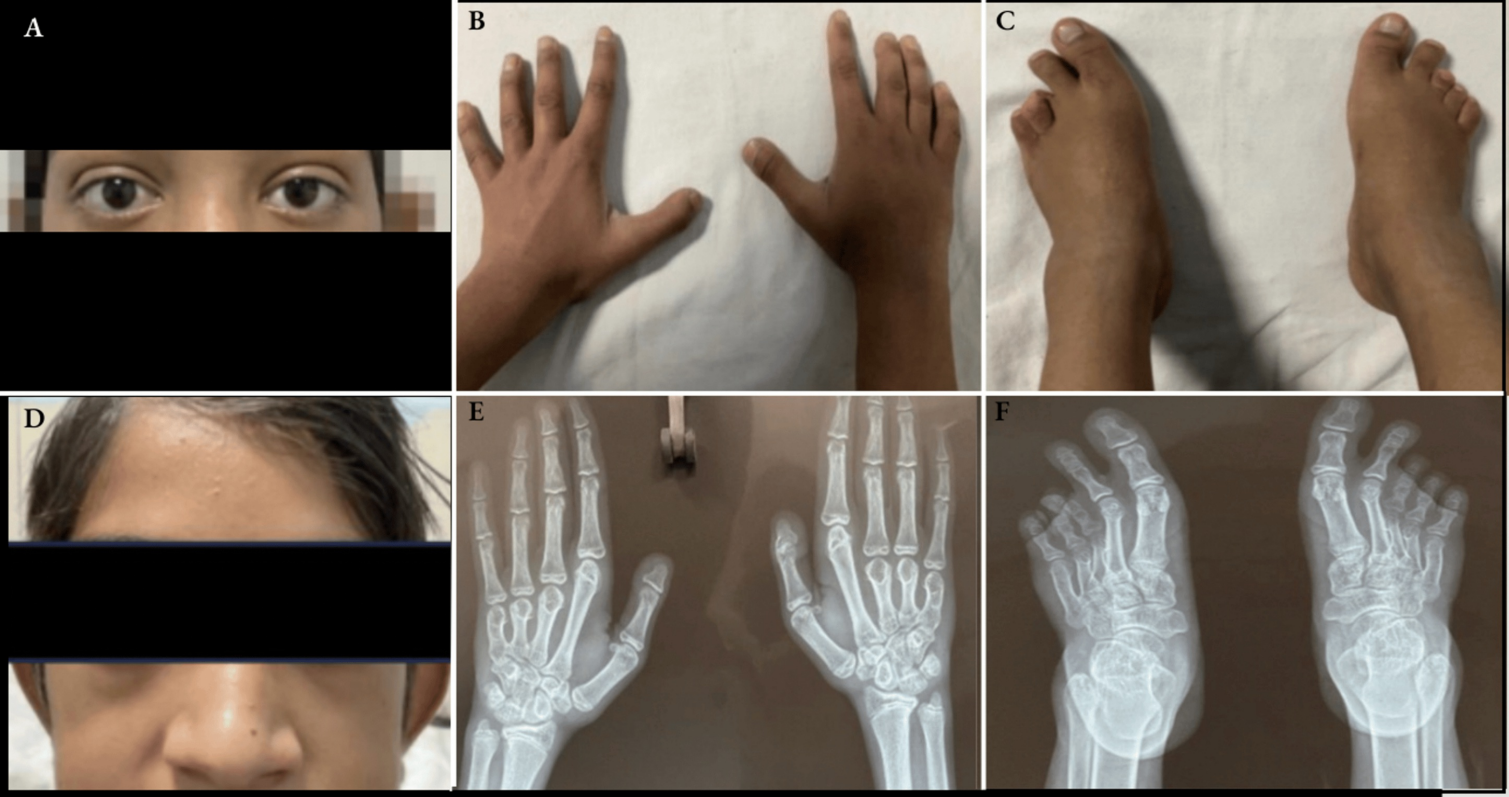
Dysmorphic and skeletal features in patient with SNAH (Panels A-F) A: Ocular telecanthus with positive Stellwag’s sign, B: Hand photograph (3rd-5th metacarpal shortening), C: Foot photograph (3rd-5th metatarsal shortening), D: Flat nasal bridge, E: Hand X-ray, F: Foot X-ray (Images have been anonymized in accordance with patient privacy guidelines). SNAH: Sporadic non-autoimmune congenital hyperthyroidism.
